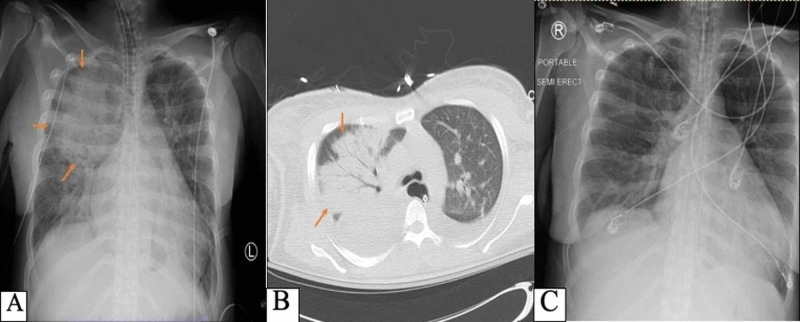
Pre- and post-mechanical ventilation chest imaging. Unilateral right upper lobe consolidation (arrows) evident on anteroposterior chest X-ray (A) and chest CT scan with contrast (B). A follow-up chest X-ray shows its resolution 24 hours after mechanical ventilation and intravenous diuretics (C). CT, computed tomography
